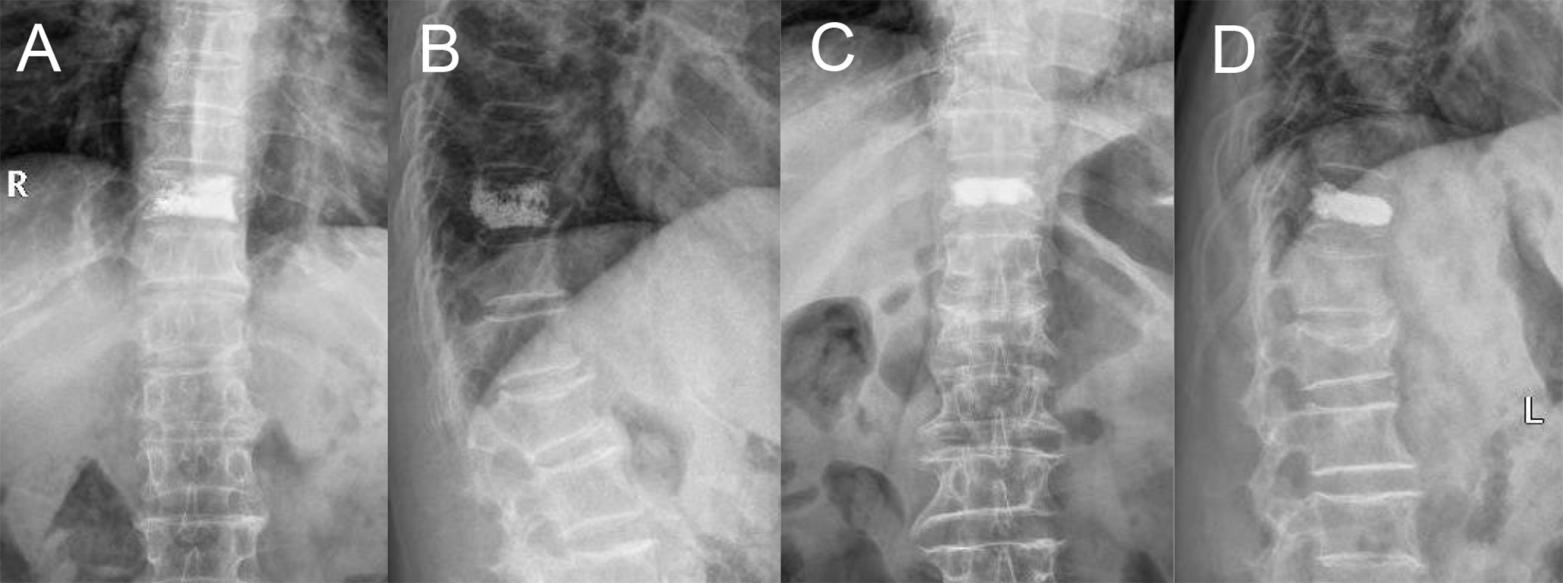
Bone cement distribution pattern on anterior and lateral radiography. (A, B) Trabecular type. (C, D) Compact type.
